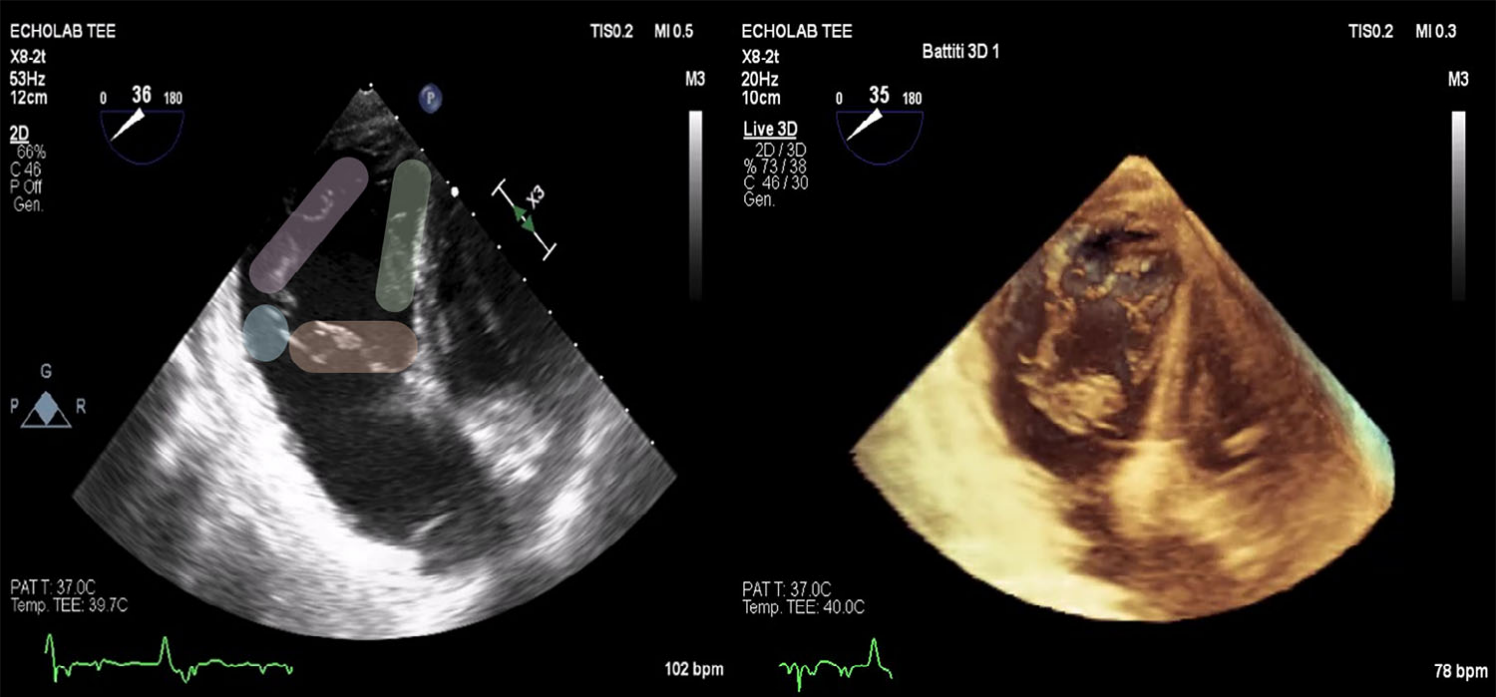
On the left: TOE TG TV oriented view: green oval indicating septal leaflet, purple oval indicating posterior leaflet, orange oval indicating anterior leaflet and blue circle indicating the antero‐septal papillary muscle. On the right: TOE TG TV‐oriented 3‐dimension live view. TG indicates trans gastric; TOE, transesophageal echocardiography; TV, tricuspid‐valve.
